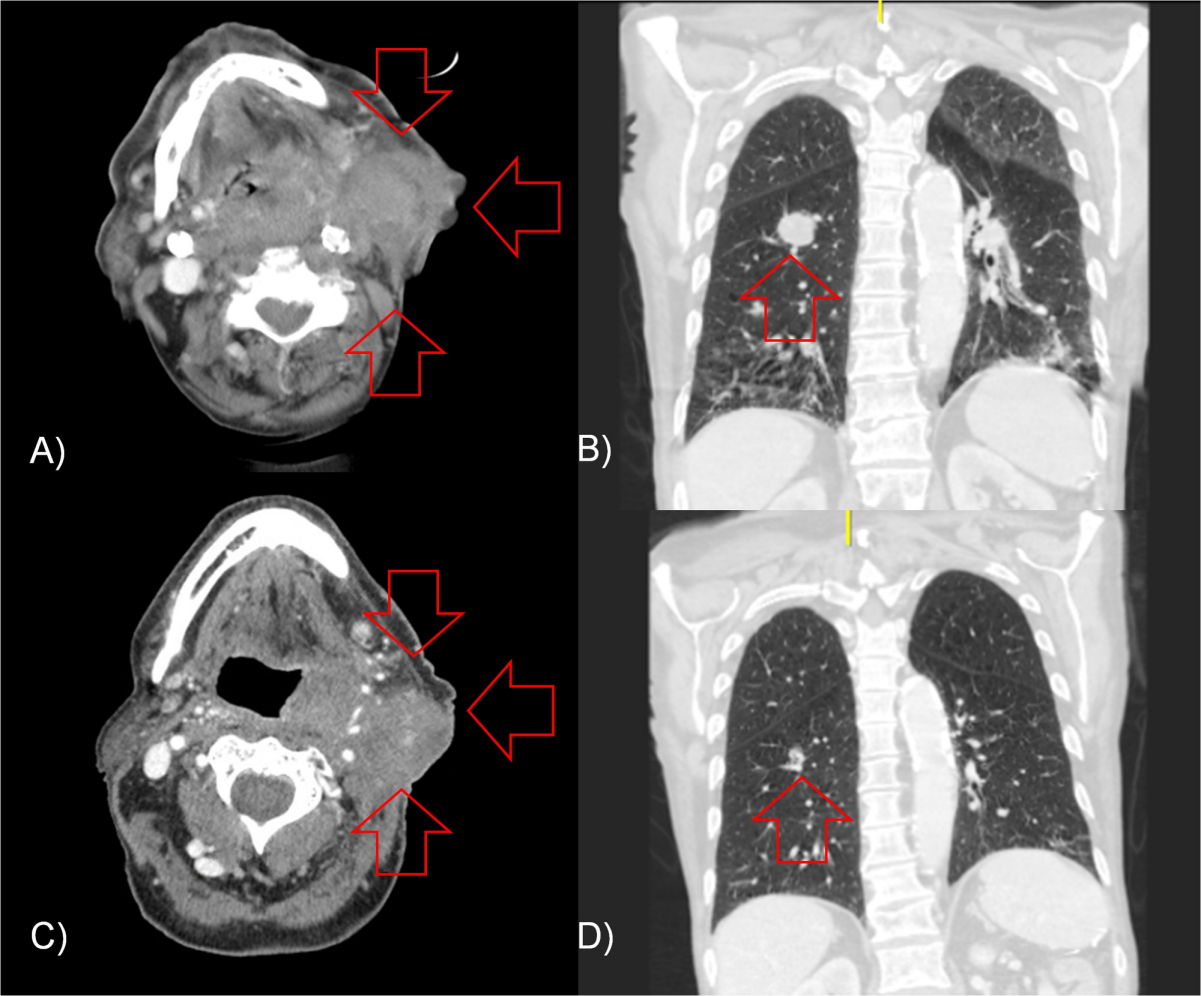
Neck and chest imaging before and after QUAD-SHOT with immunotherapy. CT imaging of the patient demonstrating (red arrows) (A) axial images of his primary disease and left neck adenopathy, and (B) coronal images of his largest pulmonary nodule, along with treatment response two weeks after completing QUAD-SHOT radiation treatment in the (C) axial and (D) coronal planes.
